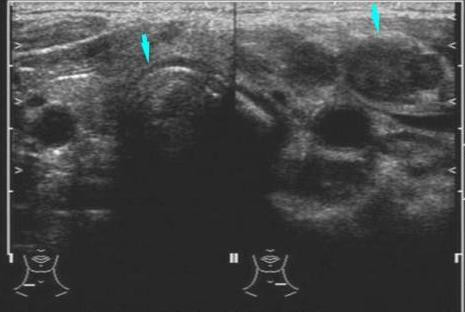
Computed tomography of the neck revealed the left thyroid tumor (arrow).
